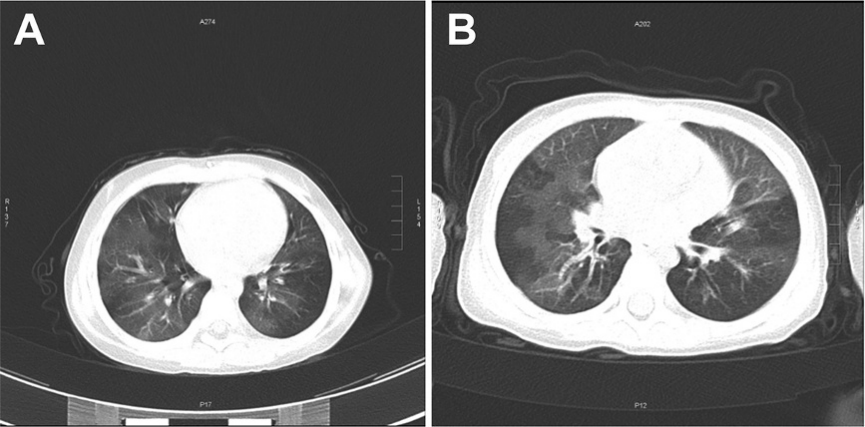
HRCT scan shows a mosaic pattern and bronchiectasis in a 2-year-old boy (A) and 3- year-old girl (B) with PIBO, respectively.
